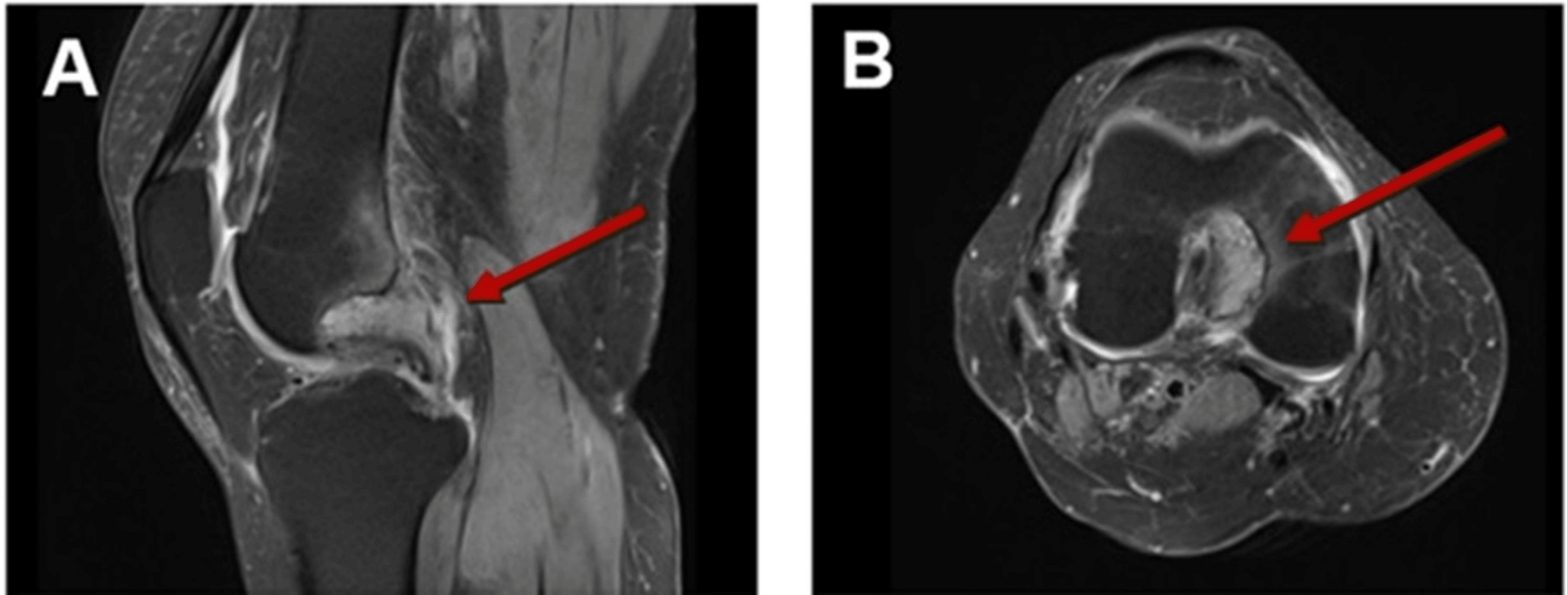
Magnetic resonance imaging without contrast of the right knee showing soft oval tissue mass in the medial intercondylar notch with adjacent bone erosion (red arrow).
